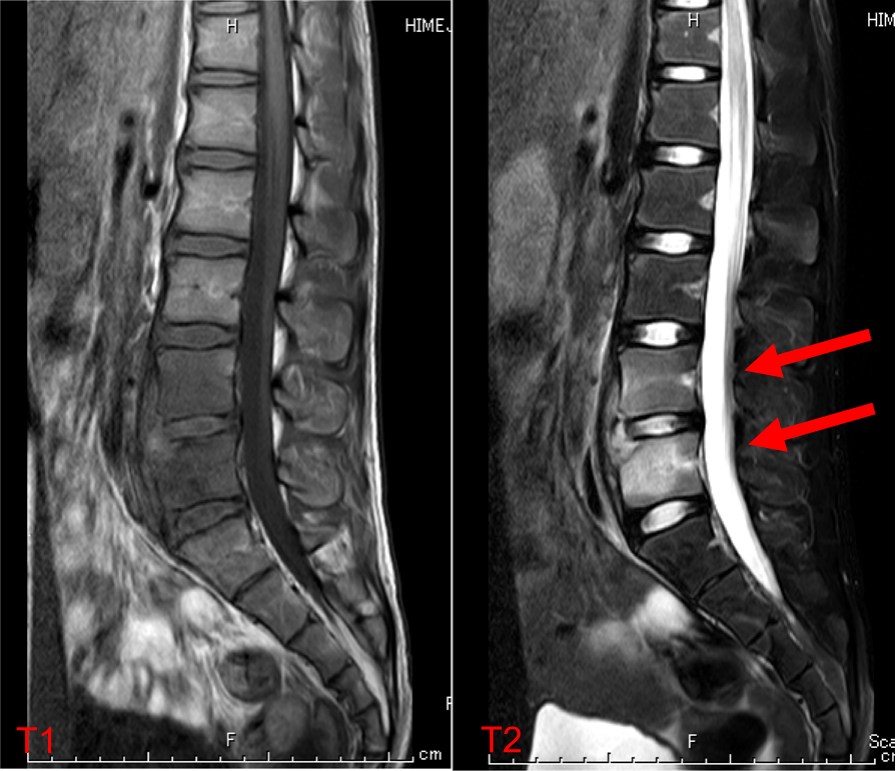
MRI on the second day of hospitalization. Abnormal hyperintensity of vertebral bodies of L4 and L5 surrounded by paravertebral soft tissue low diffusion. The interior of the spinal canal was intact
